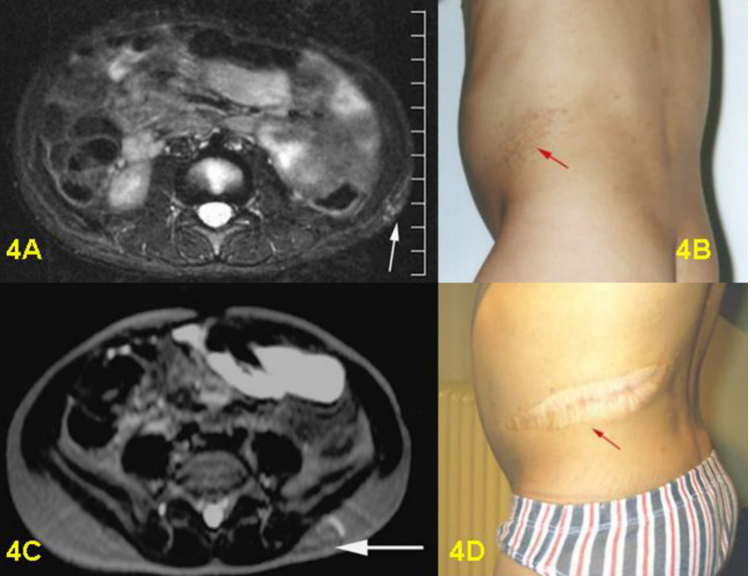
4A: Pre-surgical T2-weighted STIR-sequence, axial section: discrete signs of flat funicular, cutaneous and subcutaneous PNF near the left iliac crest with involvement of soft tissue, but without visible infiltration of abdominal muscles. 4B: Pre-surgical clinical view of PNF in the left flank. 4C: Post-surgical T2-weighted Haste-sequence: complete resection of tumor, only a smooth fibrous post-surgical induration and small scar can be identified. 4D: Post-surgical clinical view of hypertrophic scar.
